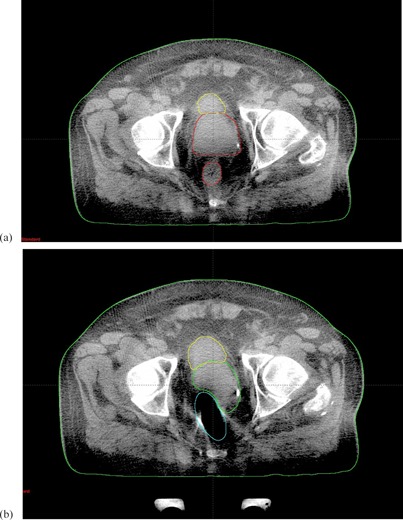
${\text{CBCT}}_{I}$ (a) and ${\text{CBCT}}_{\text{II}}$ (b) for a case of complete dislocation. The interval time was 6 min.
